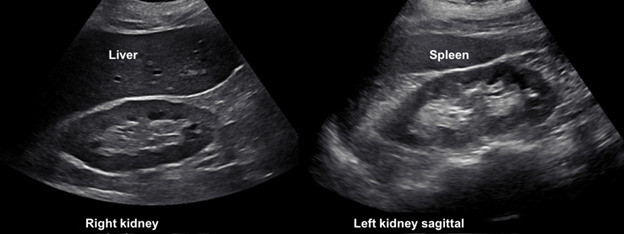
Normal kidney on ultrasound. Sagittal view of normal left and right kidney. Note the isoechoic nature of the cortex with the liver and spleen with hyperechoic sinus fat in the center. The hypoechoic medullary pyramids surround the sinus fat. Image from Koratala, A. POCUS Gallery. Renal Fellow Network. Accessed November 27, 2020. https://www.renalfellow.org/pocus-gallery/ Used with Permission
